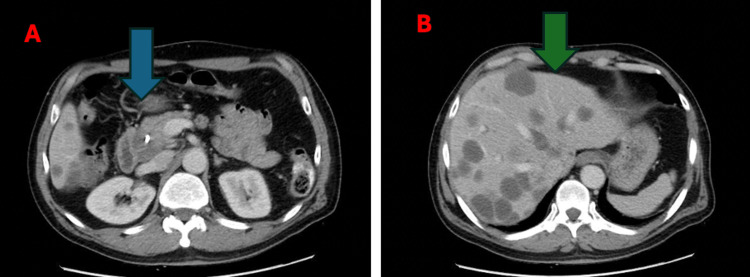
(A) CT of the abdomen shows a significant interval decrease in the size of the previously seen pancreatic mass located in the pancreaticoduodenal groove (blue arrow). (B) CT of the abdomen shows a significant decrease in the size and number of the hepatic metastatic lesions (green arrow).
